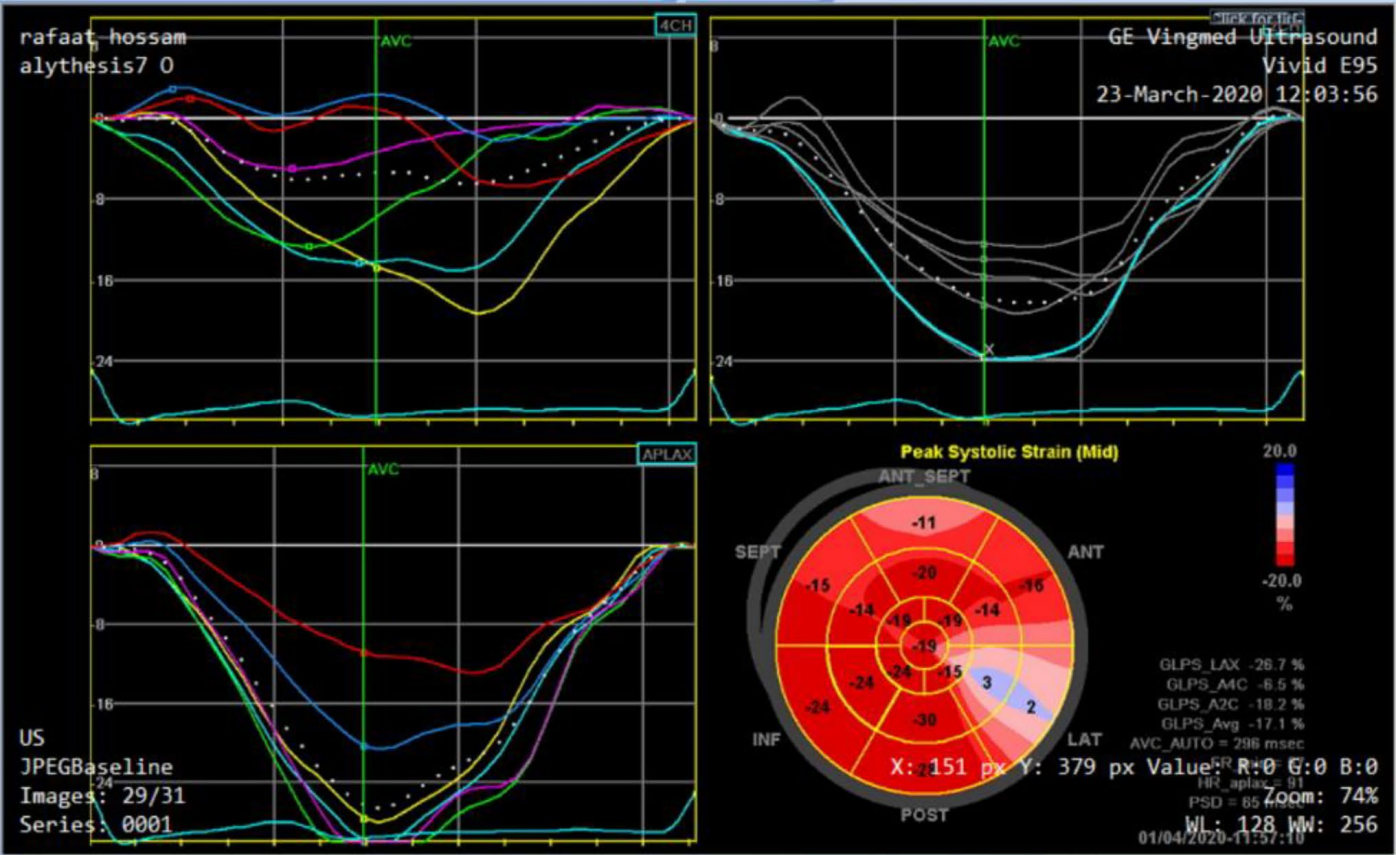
From subject No (9) showing DICOM images presenting curves of the longitudinal strain of LV at the apical 4,3 and 2 chambers views with an estimation of the global longitudinal strain and bull eye
